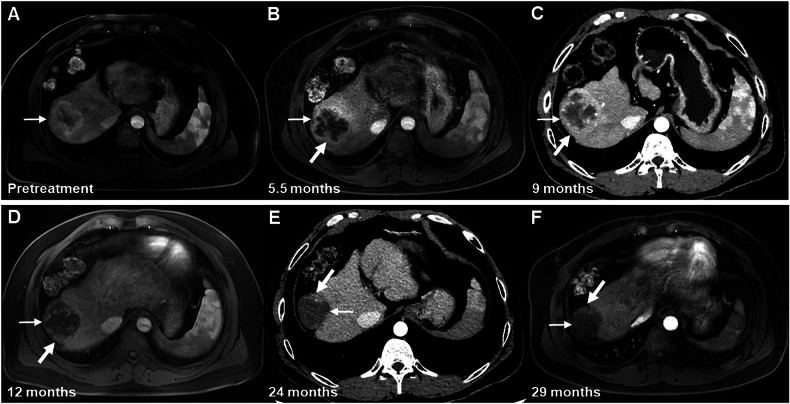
Images of a 34-year-old man with HCC treated by SIRT. MRI or CT obtained during the arterial phase. A Pretreatment contrast-enhanced MRI scan shows a 5.7 cm clinically diagnosed HCC (arrow) at segment 8 of the liver. B On the posttreatment contrast-enhanced MRI scan performed at 5.5 months after SIRT, masslike enhancement (thick arrow) was seen and decreased in size in the treated lesion (thin arrow), and this lesion was assigned to the LR-TR nonprogressing category. C–F On 9–29-month follow-up CT or MRI scans, masslike enhancement (thick arrows) was seen and further decreased in size or stable in the treated lesion (thin arrows); thus, this lesion remained in the LR-TR nonprogressing category during this period. CT, computed tomography; HCC, hepatocellular carcinoma; LR, liver imaging reporting and data system; MRI, magnetic resonance imaging; SIRT, selective internal radiation therapy; TR, treatment response
